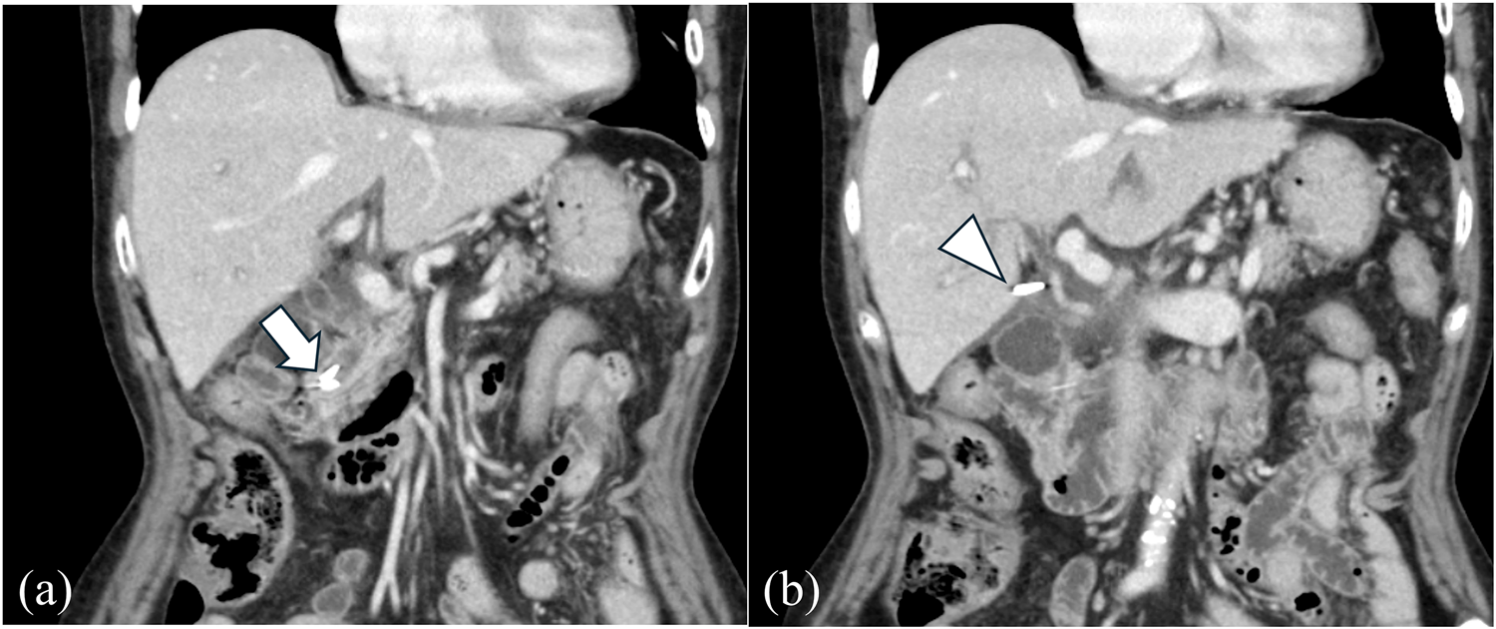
Contrast‐enhanced abdominal computed tomography (CT) scan at the time of the recent hospitalization (coronal view). (a) Two clips overlap in the lower bile duct (arrow). (b) The clip that had been closing the cystic duct on the previous CT scan was still in place (arrowhead). Figures 2 (a) and (b) are both 483 × 413 mm, 330 dpi.
